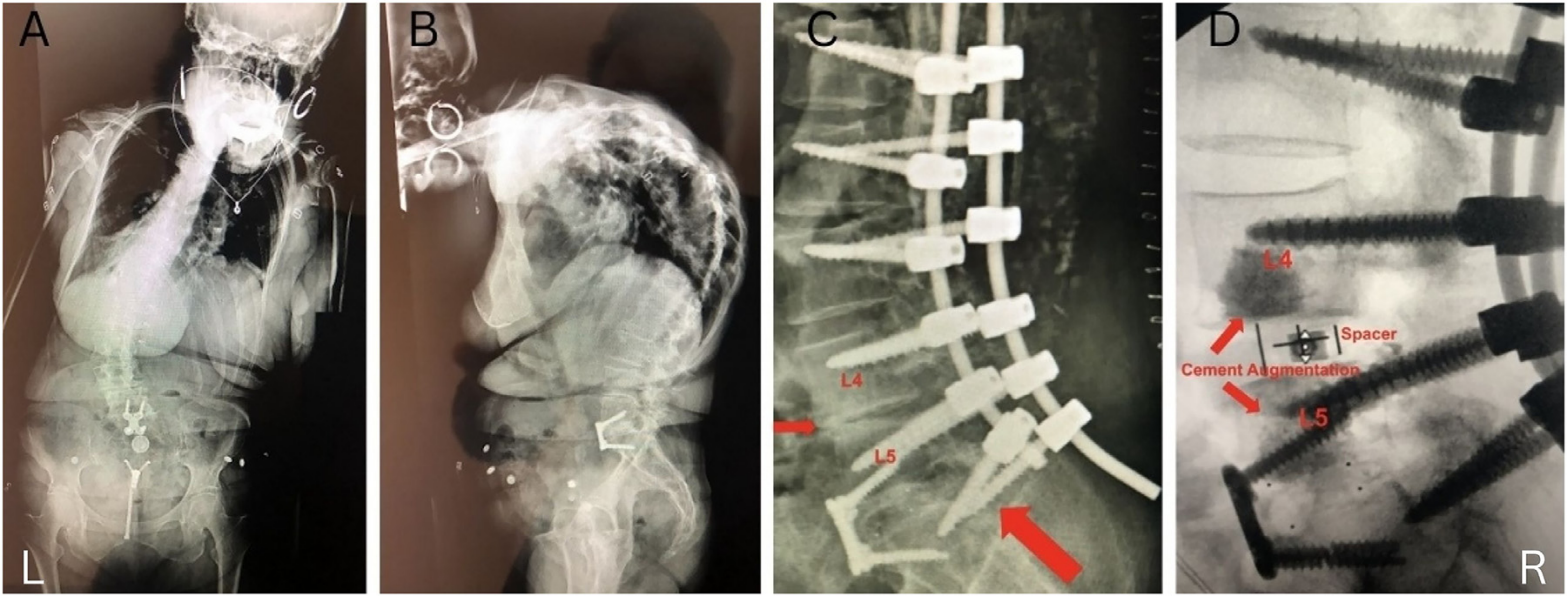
A. Anteroposterior preoperative radiograph demonstrating kyphoscoliosis. B. Lateral preoperative radiograph demonstrating kyphoscoliosis. C. Lateral radiograph demonstrating loosening and migration of L5, S1 pedicle screws and disc collapse and kyphosis at L4–L5. D. Lateral intraoperative radiograph demonstrating cement and spacer placement.
